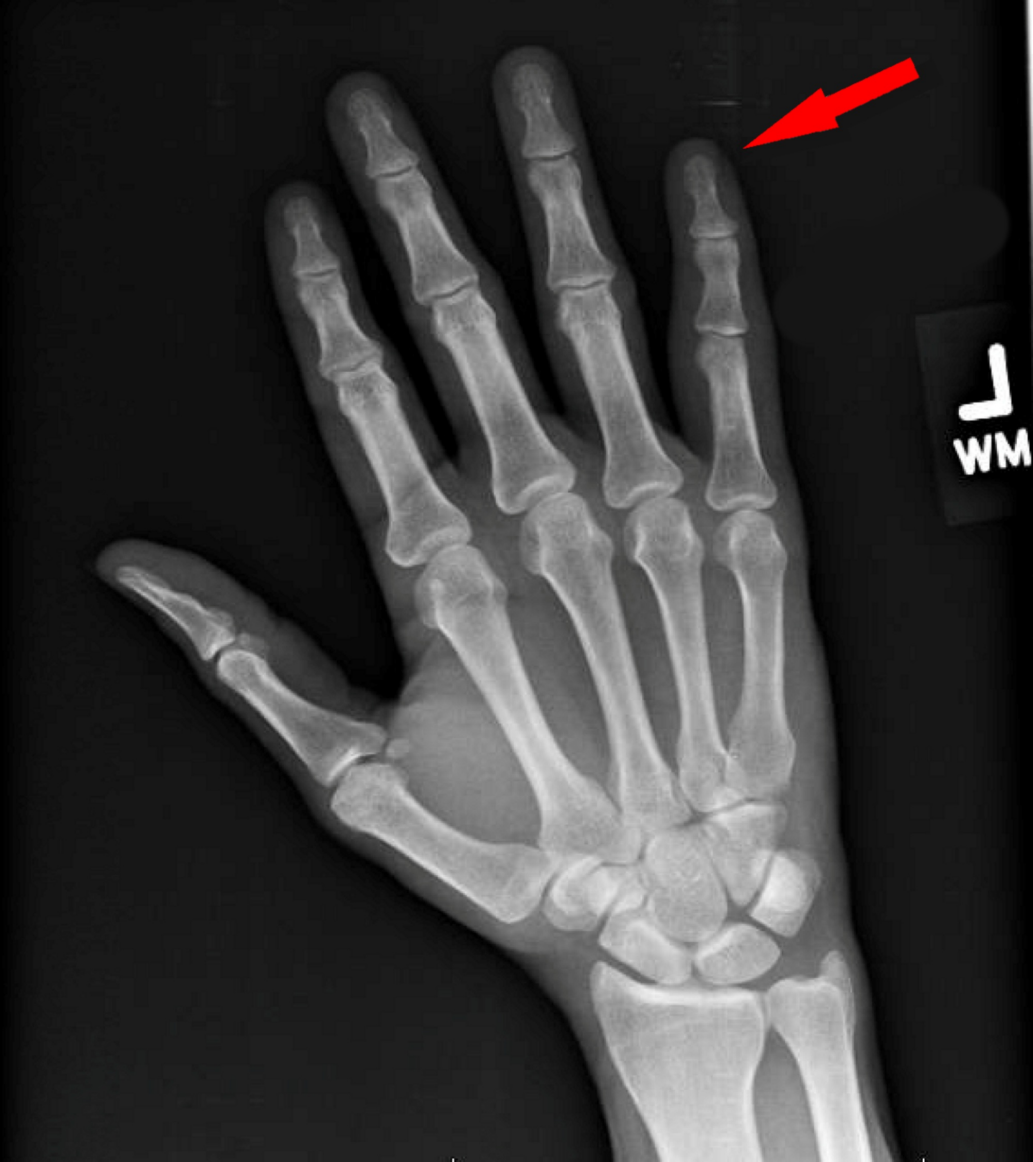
X-ray dorsal view of the left hand immediately following crush injury.
